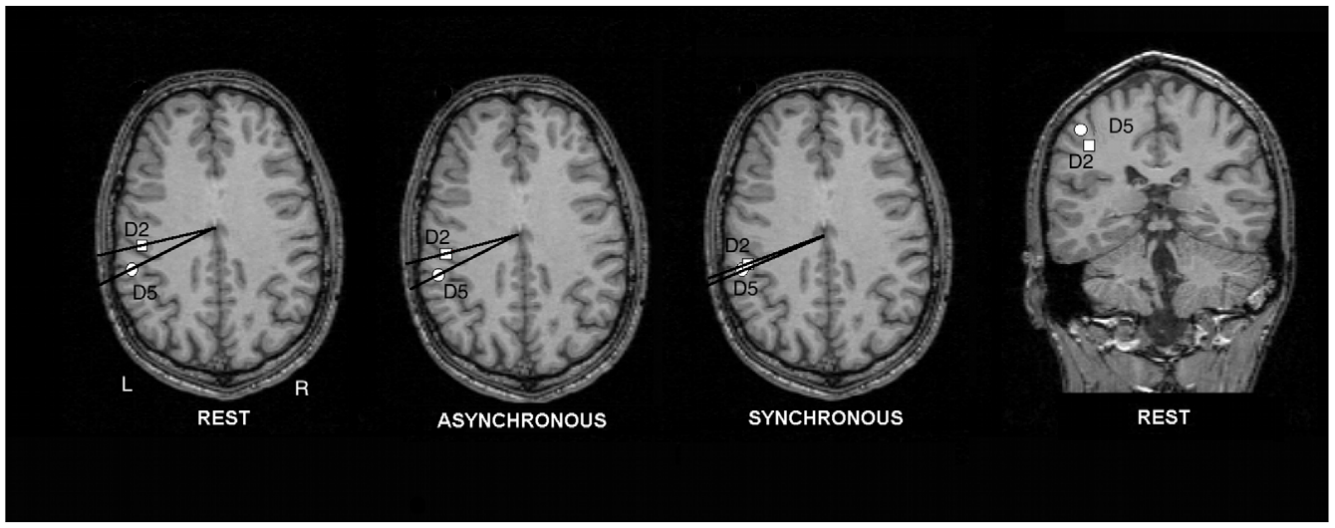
Dipole localizations of the SEFs for one representative subject overlaid onto an axial MRI slice. The positions of the dipole sources are specified in polar coordinates. The squares depict the cortical representations of D2; the circles show the representations for D5. Note the differences in polar angle Δφ between rest, asynchronous, and synchronous brushing, pointing to a decrease of the cortical distance in the synchronous brushing condition. For verification of dipole sources a coronal slice (rest condition) is depicted.
